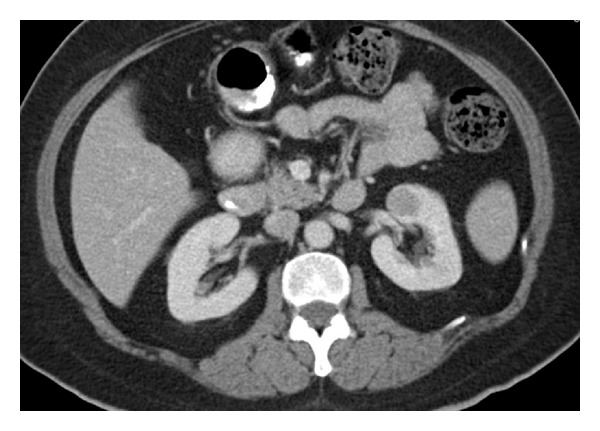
Contrast-enhanced CT showing a well-defined hypodense mass at the anterior midpole of the left kidney.
